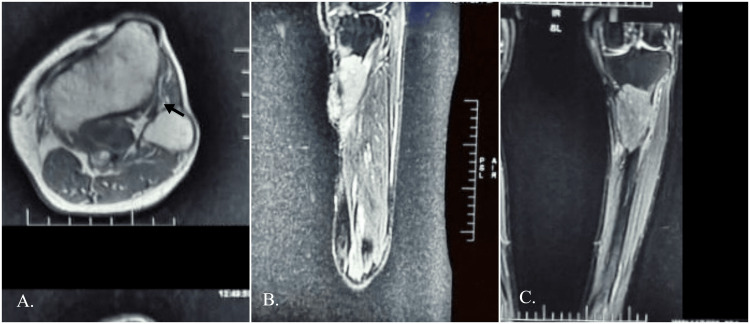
MRI sections showing lytic lesion in the proximal tibia A: Axial view shows the tumour confined to the tibia with an intact interosseous membrane (black arrow); B: Sagittal view, C: Coronal view
